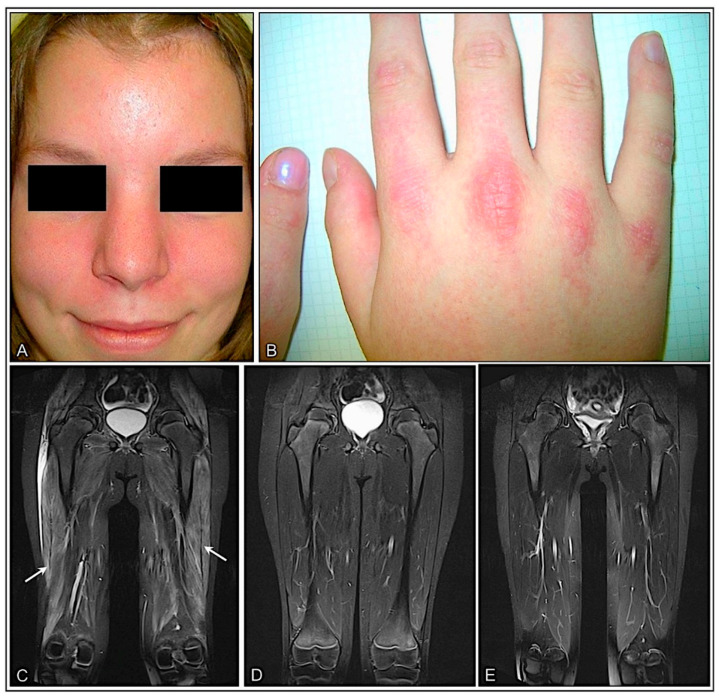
Proximal myopathy and skin lesions are the clinical hallmarks in patients with jIIM. Mild facial erythema (A) and discrete erythematous papules overlying the metacarpal joints (Gottron’s papules) (B) in a 16-year-old girl with DM (P5). Coronal MR images in a 10-year-old patient with anti-NXP2 antibody-associated DM (P4) showing mild contrast enhancement in T1-weighted images and diffuse oedema of the thigh muscles at first presentation (arrows) (C) resolving completely with treatment after 6 (D) and 12 months (E).
